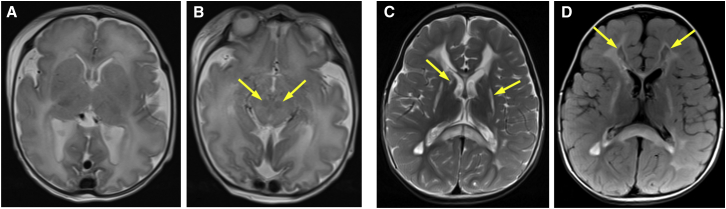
Neuroimaging of Subjects 1 and 2 (A and B) Axial T2 imaging of subject 1 shows diffusely abnormal hyperintensity of the entire white matter and decreased cortical folding for her age (A). Signal abnormalities within the brain stem and strikingly low signal affecting red nuclei (arrowheads) are also apparent (B). (C) Axial T2 imaging of subject 2 shows extensive signal abnormalities of the cerebral white matter, including the corpus callosum. In addition, basal ganglia are affected, especially the putamen and caudate nucleus (arrowheads). (D) Axial FLAIR imaging of subject 2 shows partial cystic degeneration of the affected cerebral white matter (arrowheads).
